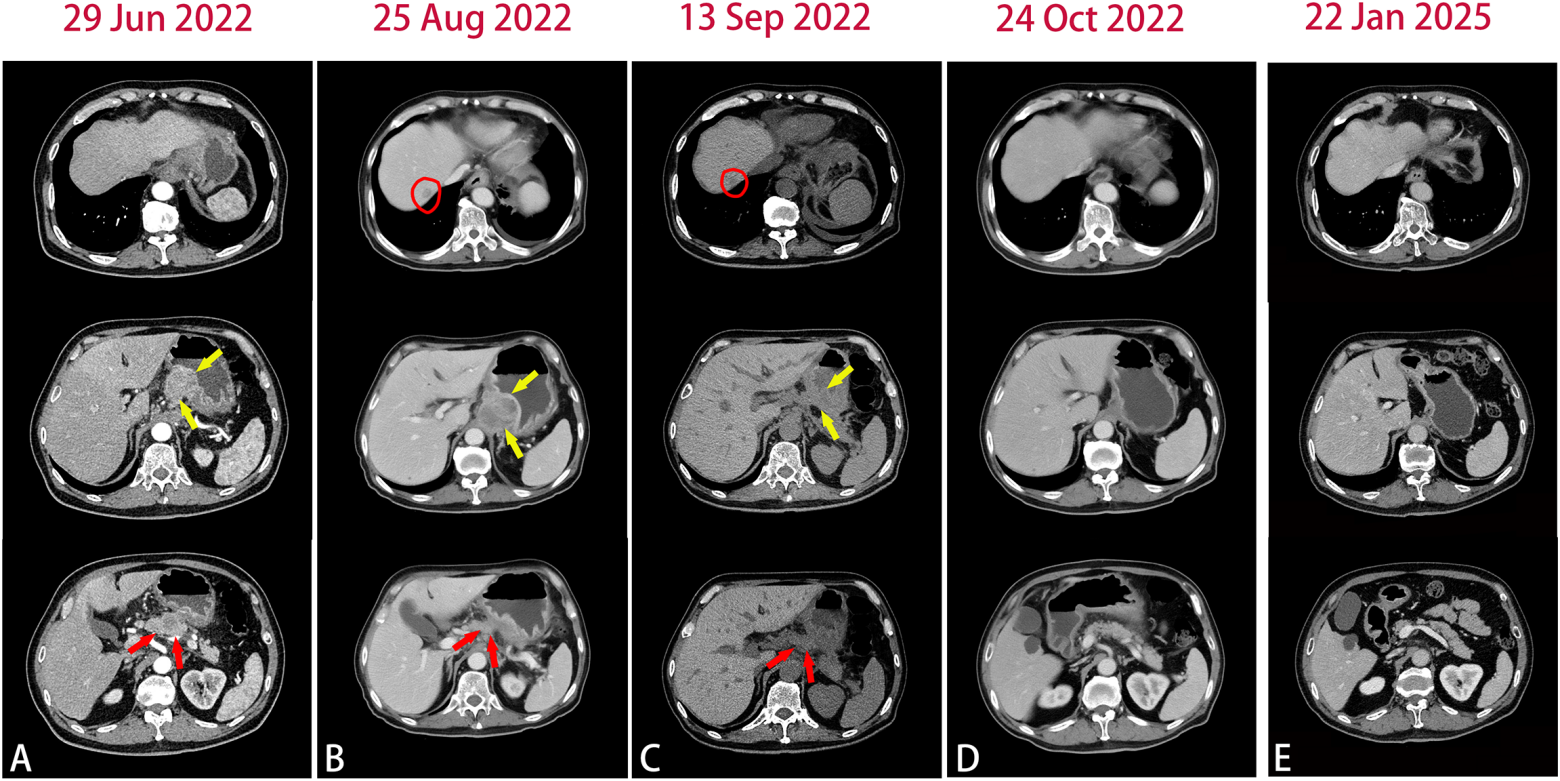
Abdomen CT images in the course of disease. (A) Baseline CT images showed the primary lesion (yellow arrow) and pancreas involvement (red arrow). (B) After 2 cycles of chemotherapy plus pembrolizumab, CT images showed newly emerged liver metastatic lesion(circle) and the primary lesion(yellow arrow) were slightly enlarged. (C) CT images after tocilizumab treatment. (D) After 2 cycles of second-line chemotherapy plus pembrolizumab, CT images showed significantly shrunk primary lesion and liver metastases. (E) The most recent CT images.
